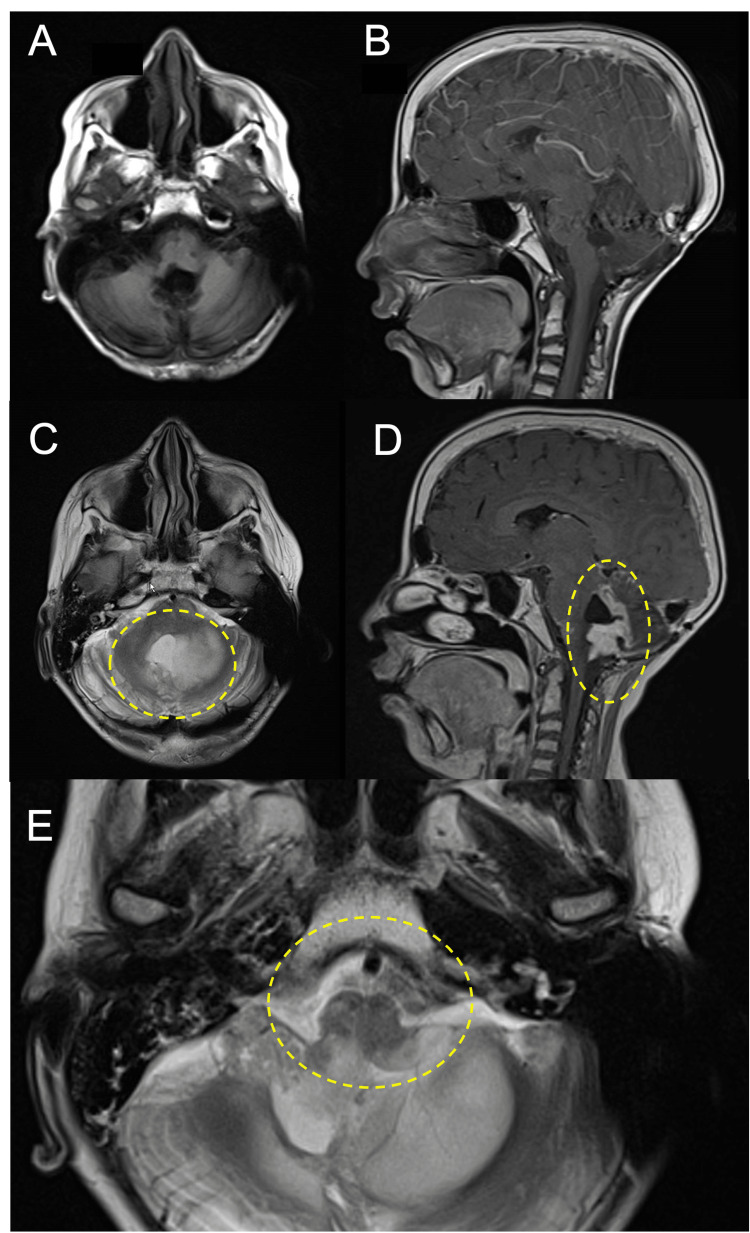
Comparative MRI imaging of the brain in a patient with medulloblastoma. a.) Axial and b.) sagittal gadolinium contrast images five years and eight months following completion of therapy without evidence of recurrence. c.) Axial and d.) sagittal gadolinium contrast images at the time of relapsed medulloblastoma showing enhancing nodular relapsed tumor extending from the fourth ventricle into the left cerebellum. There is extensive leptomeningeal enhancement extending from the fourth ventricle to the right cerebellopontine angle, into the right internal auditory canal, and along the right tentorium as well as enhancement along the right anterior medulla and the midbrain bilaterally. e.) Enlarged axial T2 sequence demonstrating posterior medulla involvement of the recurrent tumor.
